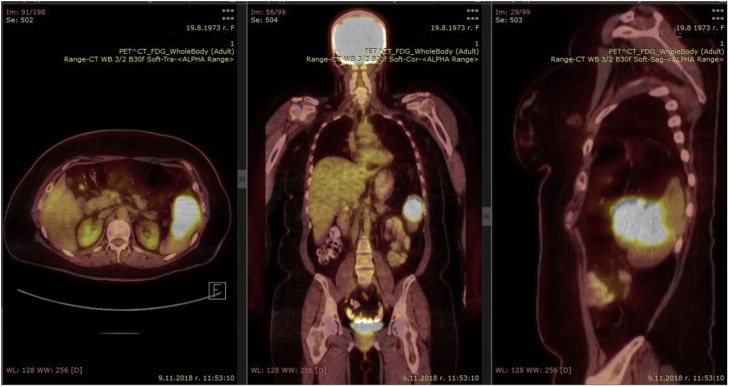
PET/CT of patient with spleen metastasis from ovarian cancer – case 1.
